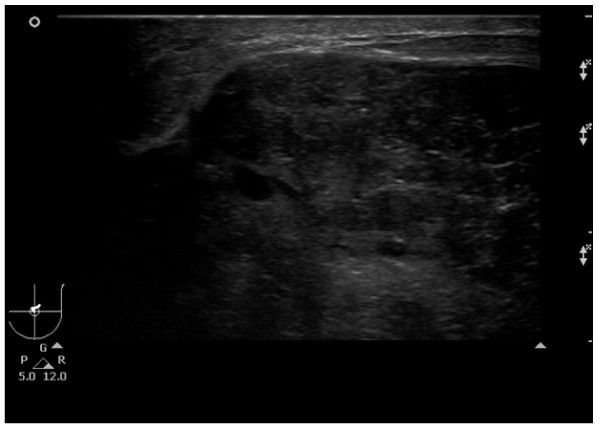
Ultrasound of benign phyllodes tumor in left breast.
